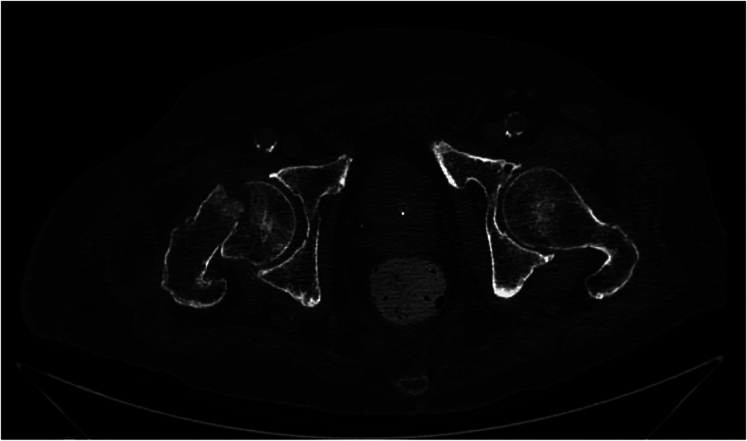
Subcapitate fracture axial CT showing decomposition.
